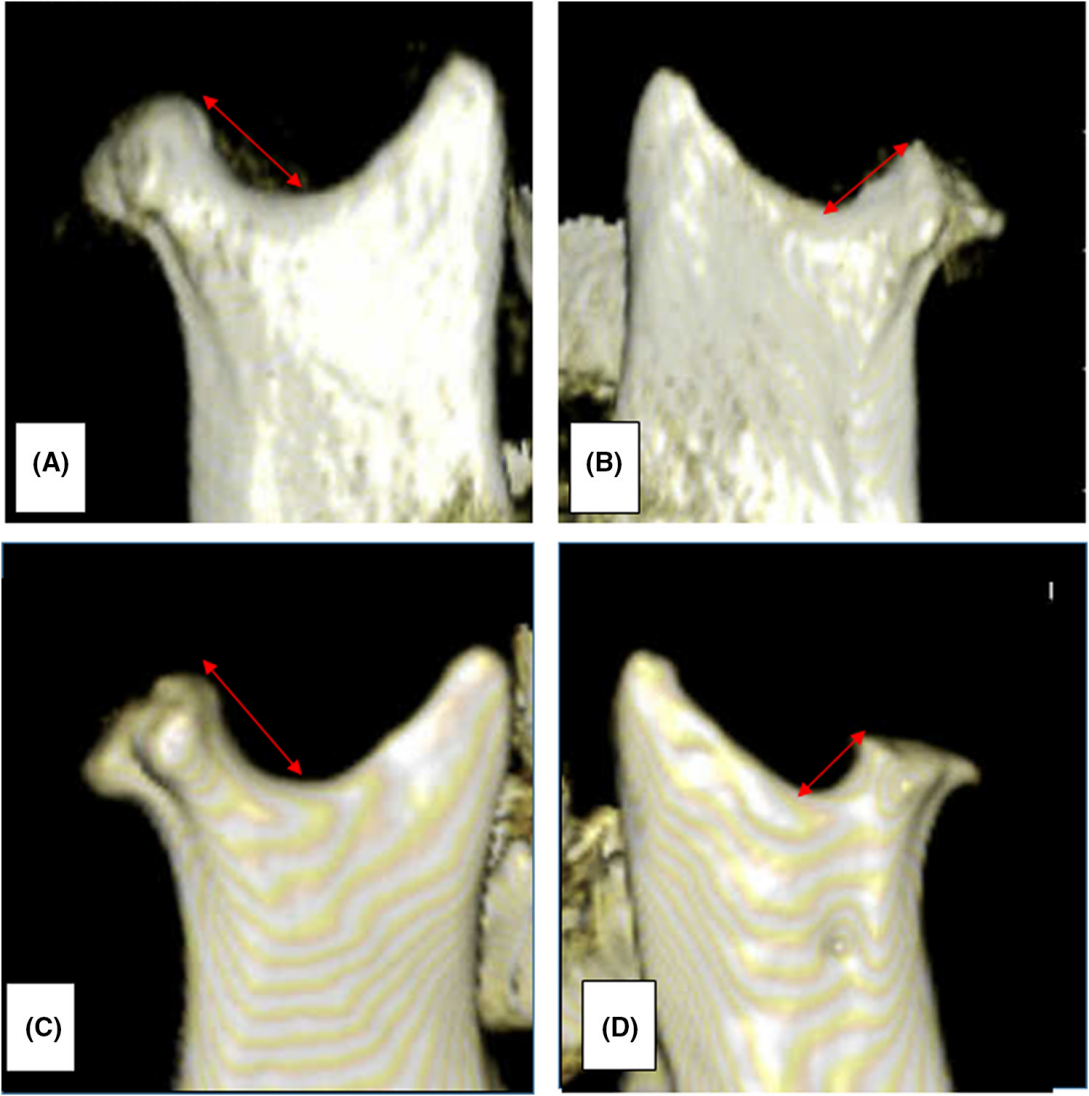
3D‐CT image of the mandibular condyle. (A): Preoperative right mandibular condyle (March 7, 2013) (B): Preoperative left mandibular condyle (March 7, 2013) (C): Right mandibular condyle 1 year and 3 months after LF + HS and MDO and 11 months after GP (August 14, 2014). (D): Left mandibular condyle 1 year and 3 months after LF + HS and MDO and 11 months after GP (August 14, 2014).
